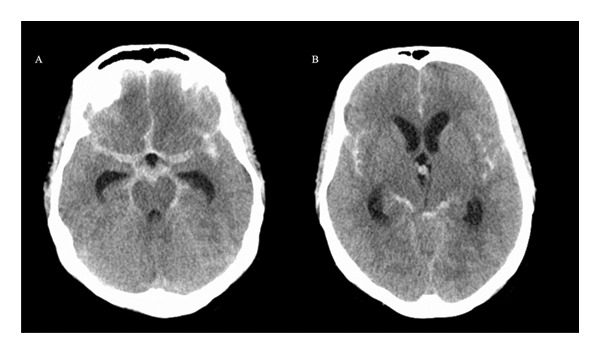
(A, B) Noncontrast axial brain CT performed on Day 1. (A) Extensive subarachnoid hemorrhage (SAH) within the basal cisterns and a distended temporal horn of the lateral ventricle. (B) SAH within the basal cisterns and bilateral sylvian fissures, and distended frontal and occipital horns of the lateral ventricle.
